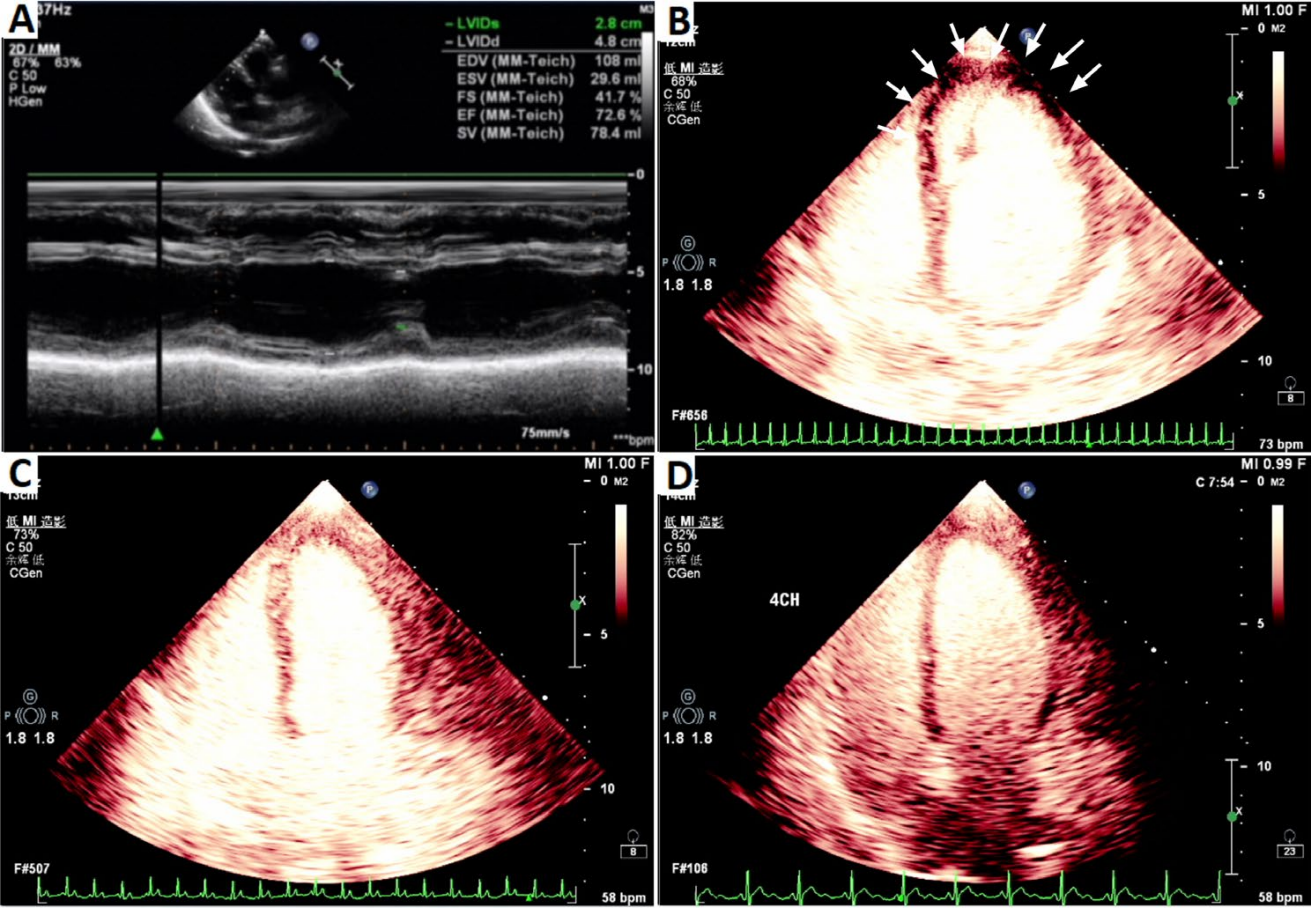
Normal echocardiography at admission (A). LVO on Day 1 after PCI shows the middle and lower segments of the ventricular septum, the anterior wall of the left ventricle, and the ventricular wall of the apical segment (arrow) at the onset of Takotsubo syndrome (B). On day 7 after PCI, only slight akinesia with a normal ejection fraction of 65% can be seen (C). Normal LVO at 2 months later (D)
